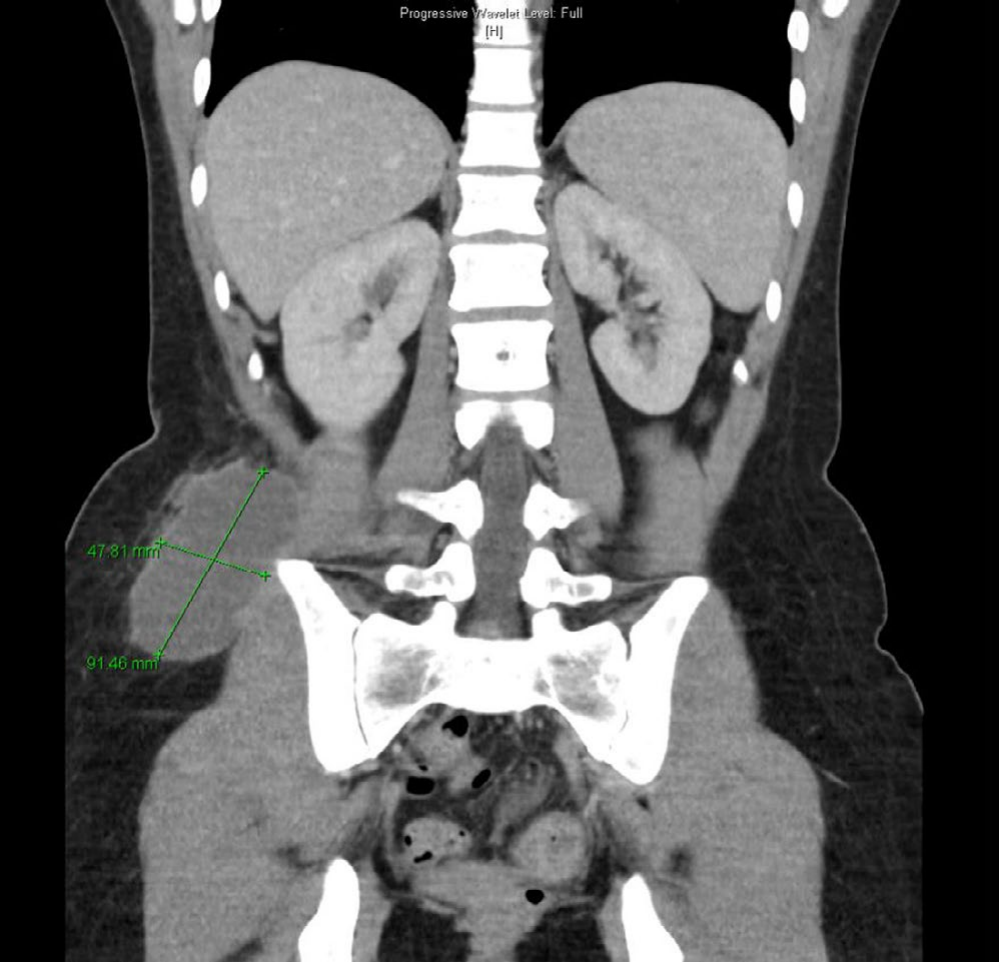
Computed tomography abdomen showing right lateral abdominal wall collection
